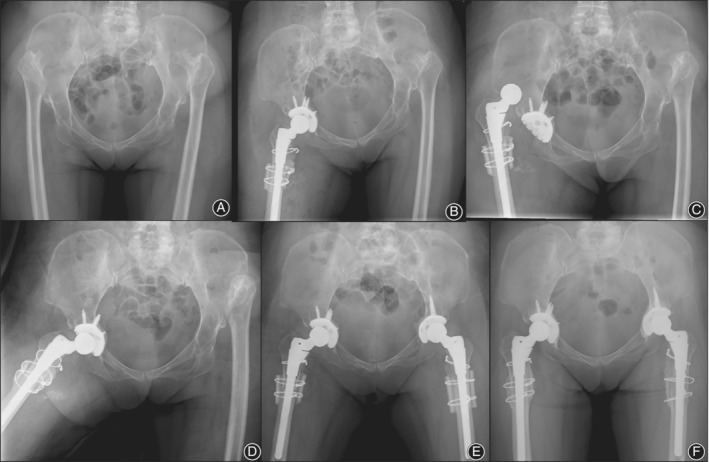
Radiographs of a 66‐year‐old woman with bilateral Crowe type IV DDH. (A) preoperative radiographic image showing high dislocation of both hips without a false acetabulum. (B) Radiographic image at post‐operation day 1. THA with 28‐mm metal femoral head performed in the right hip. (C) Dislocation occurred at post‐operation day 9. (D) Closed reduction was performed followed by abduction bracing for 3 months. (E) Postoperative radiographic image showing THA combined with transverse osteotomy in the left hip. (F) At 8‐year follow‐up, no more dislocation occurred and no loosening of components was identified.
